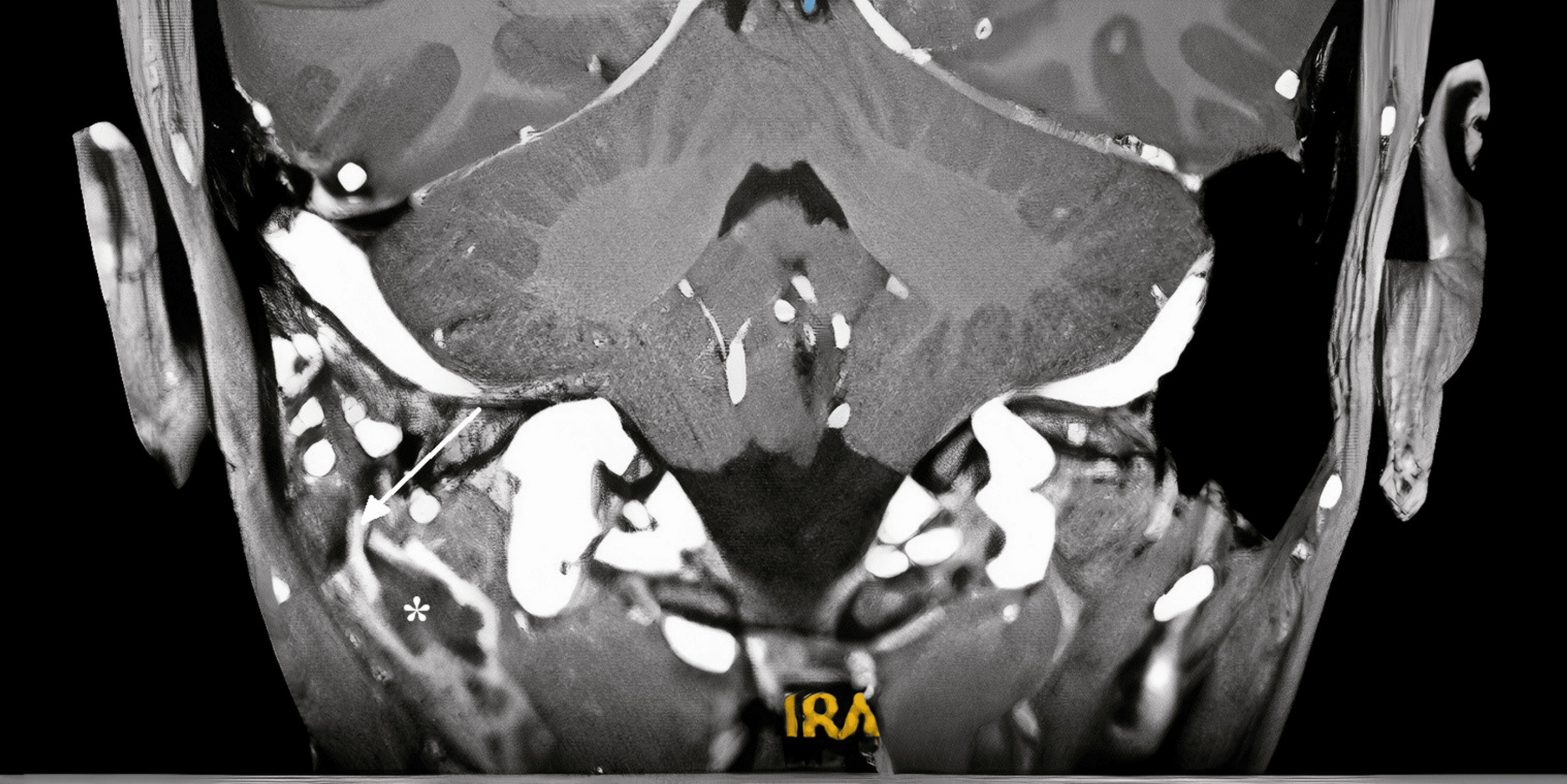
Contrast-enhanced MRI neck (T1 weighted, coronal view) Rim-enhancing lesion (*) over the right deep neck muscle adjacent to the eroded mastoid tip (arrow)
